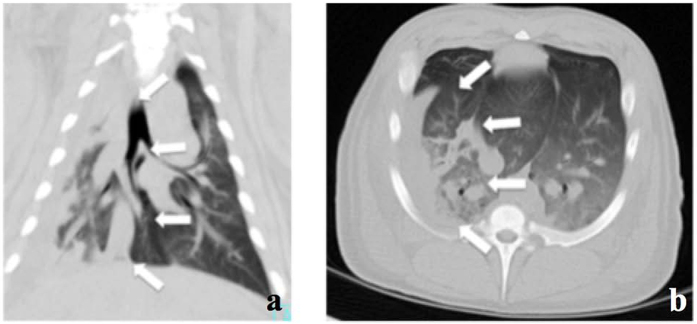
(a) CT of right lung (coronary view) with signs of lung contusion (white arrows). (b) CT of right lung (horizontal view) with signs of lung contusion (white arrows).
